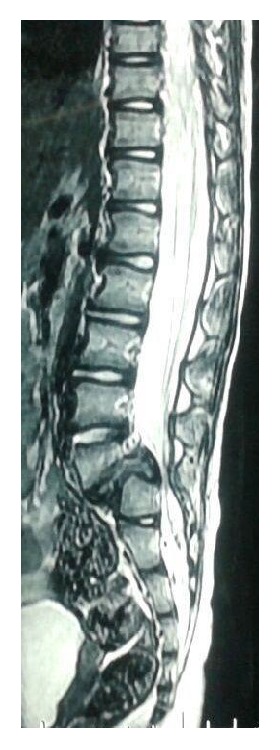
Sagittal T2 weighted MRI image.
